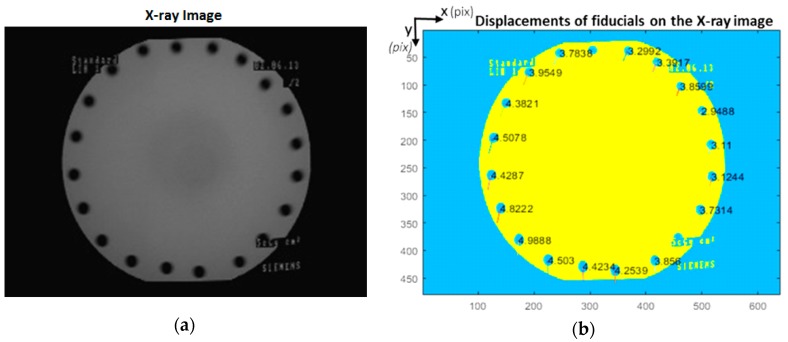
(a) The No.6 X-ray image, (b) The marker displacements in pixels (multiplied by 5 for visualization of the displacement vectors) of the No.6 X-ray image.
